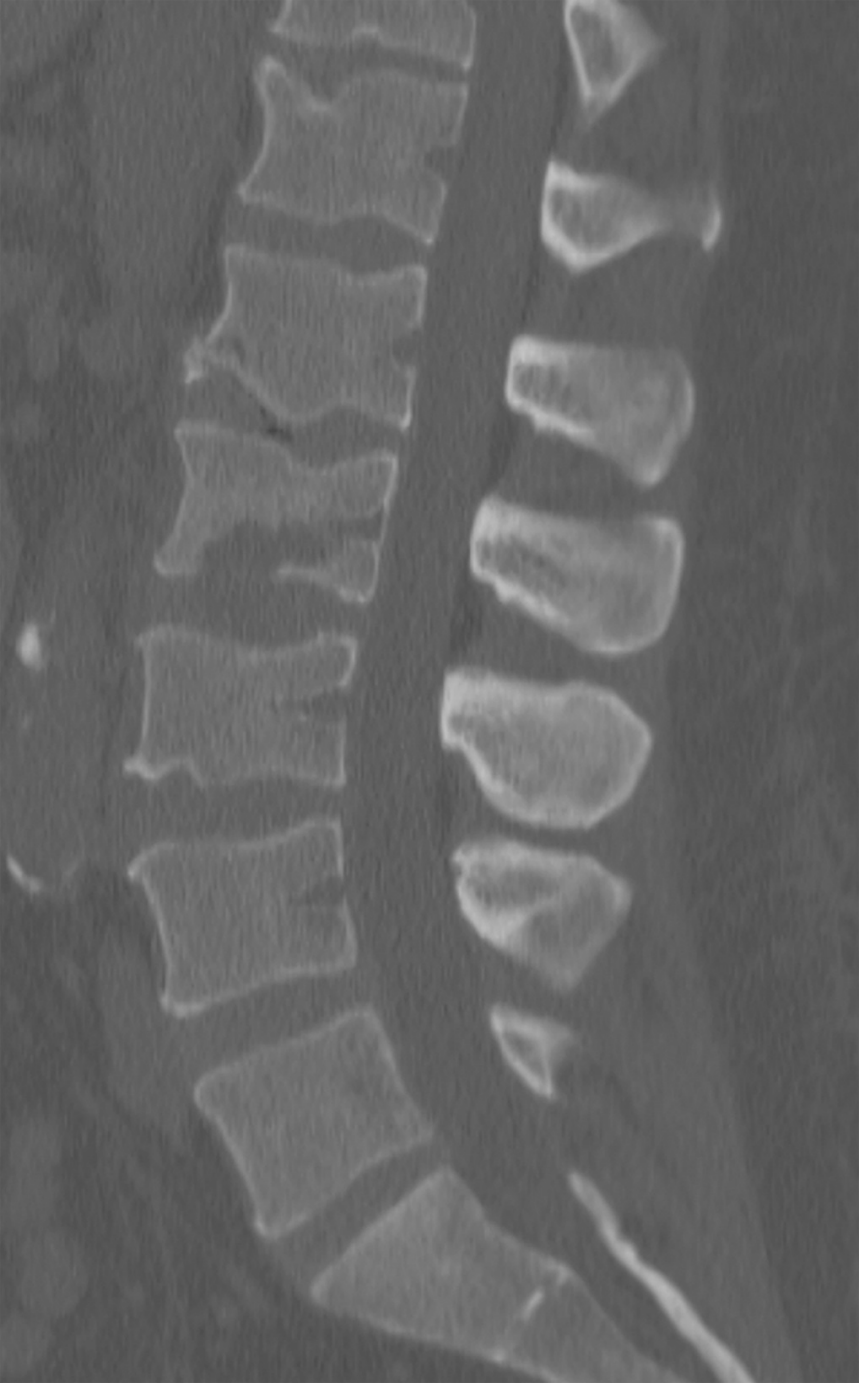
Follow-up CT demonstrating a significant progression of osseous destruction of the L2 vertebral body.
